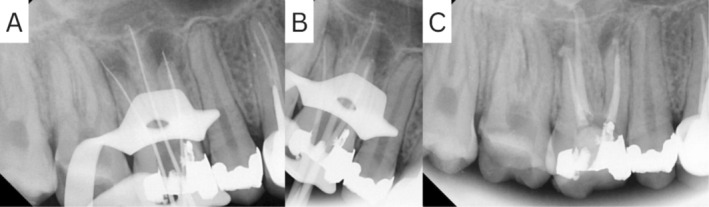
Retreatment radiographs: (A) working‐length confirmation; (B) master‐cone fit confirmation; (C) obturation (final radiograph).
